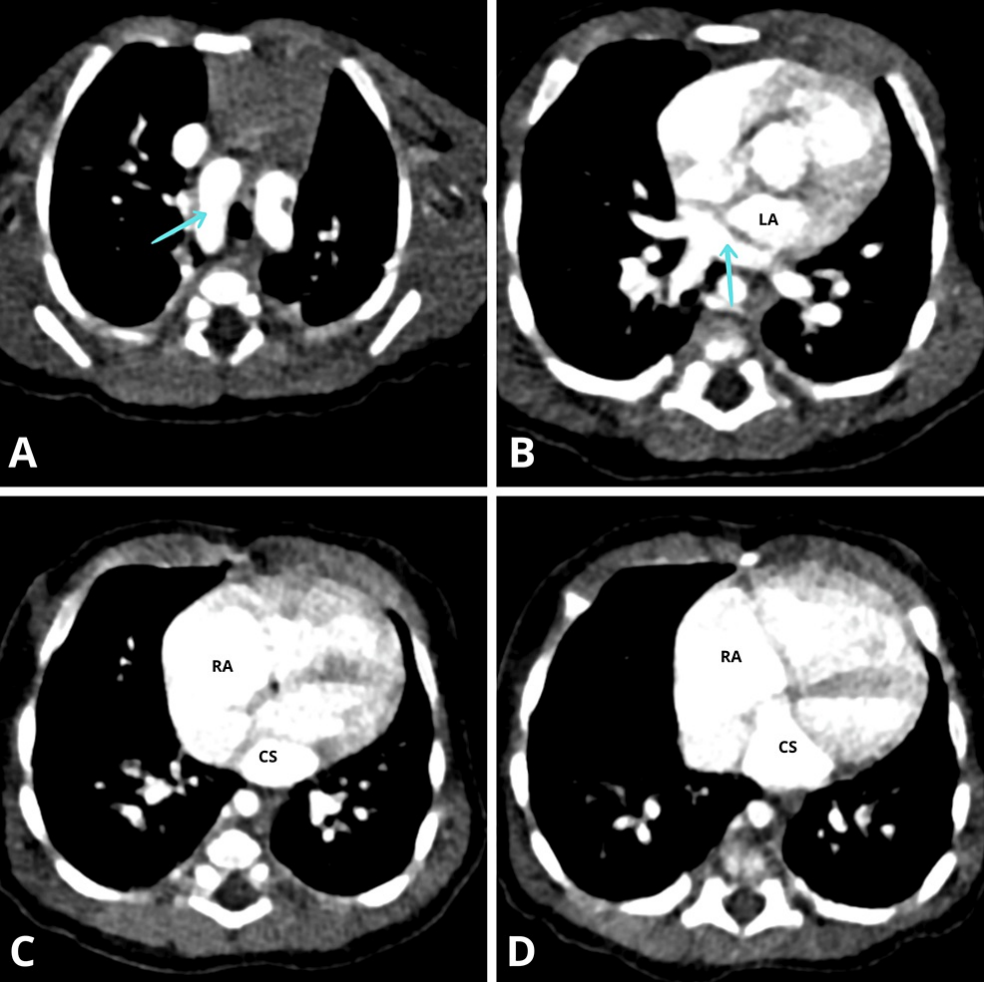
Thoracic axial CT angiography shows (A) dextroposition of the aorta (arrow); (B) retroauricular collector where pulmonary veins drain (arrow); (C) coronary sinus where the collector drains; (D) The coronary sinus draining into the right atrium. LA: left atrium; RA: right atrium; CS: coronary sinus
